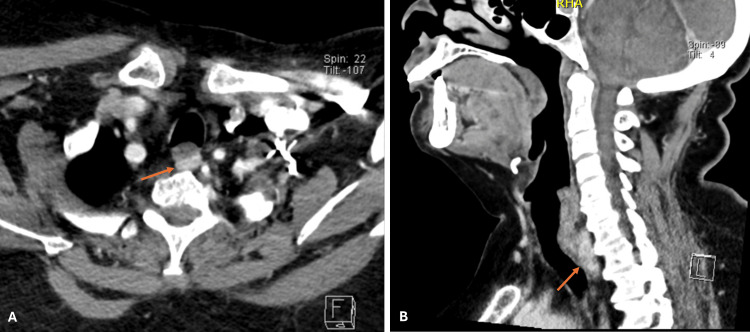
Preoperative four-dimensional neck CT scan in axial (panel A) and sagittal views (panel B) showed a hyper vascular retro-esophageal nodular lesion measuring 31x14x7 mm (orange arrow).
